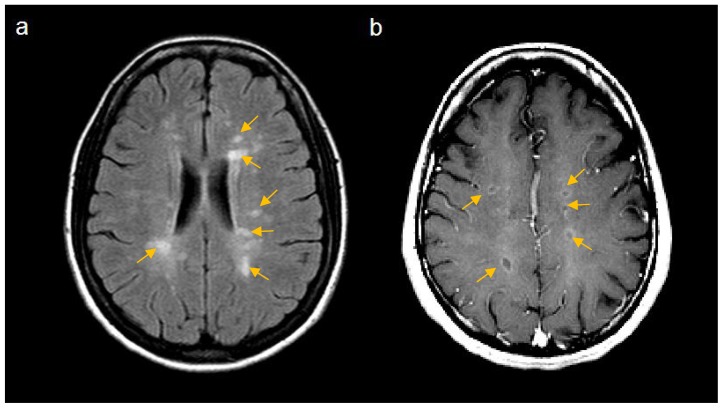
Axial magnetic resonance imaging showing (a) hyperintense lesions (arrows) on T2 weighted images and (b) ring, gadolinium (GAD)-enhancing lesions (arrows) on T1 weighted images.
